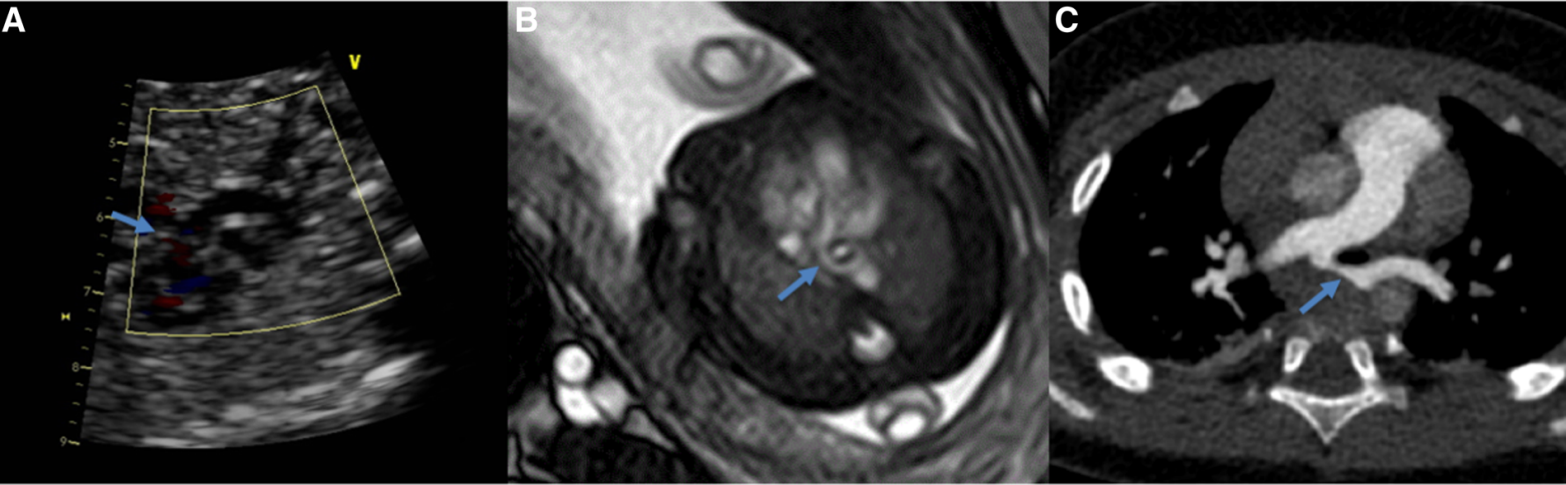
Comparing of the pulmonary artery sling obtained at ultrasound (A), 1.5T MRI (B) and postnatal CTA (C) (same fetus). The blue arrow show the left pulmonary artery.
